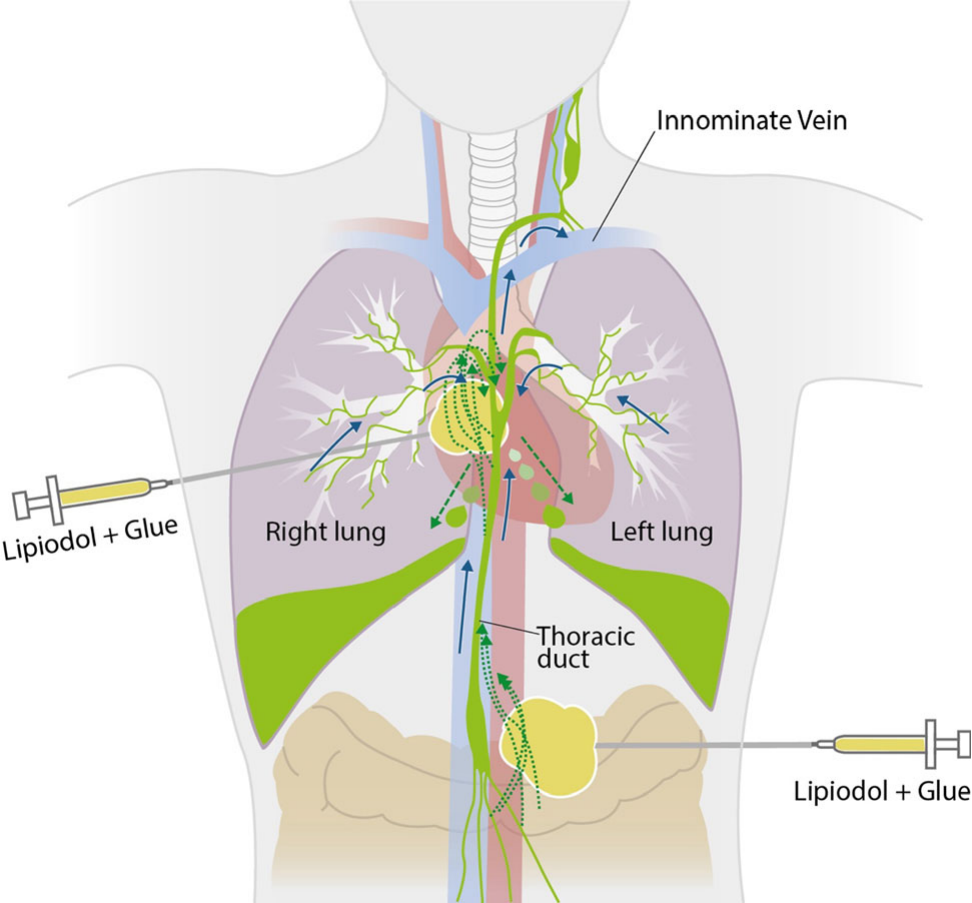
Blue arrows indicate normal lymphatic flow. Chaotic and disrupted lymphatic channels (dotted green arrows) within lymphoma bulks lead to abnormal lymph accumulation (dashed green arrow) and chylothorax. Puncture of the lymphoma bulks under CT guidance and injection of the lipiodol-glue mixture
